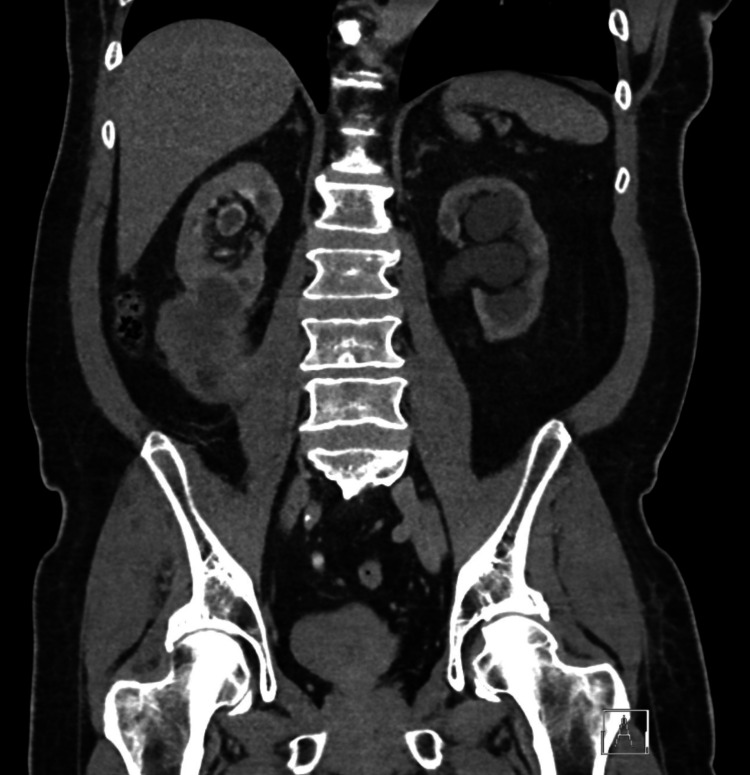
Primary right lower pole renal tumor with involvement with right iliopsoas musculature with filling defect in the superior pelvis on CT urogram CT: computed tomography
